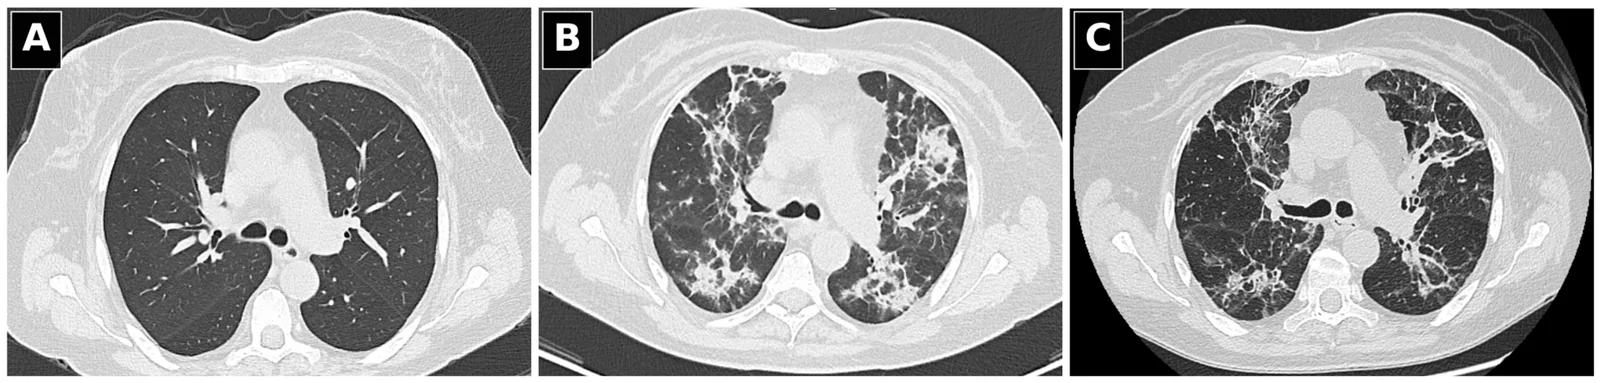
Serial axial chest computed tomography at the level of the tracheal bifurcation, lung window. (A) On 28 July 2025, before starting imatinib: normal lung parenchyma, with no interstitial or airspace abnormality. (B) On 30 December 2025, approximately five months after starting imatinib and one month after symptom onset: extensive bilateral peribronchovascular consolidative opacities with irregular margins, predominantly in the upper lobes, without pleural effusion, in an organizing-pneumonia-like distribution. (C) On 11 February 2026, at the time of acute respiratory failure: coarse reticulation with interlobular and intralobular septal thickening, traction bronchiectasis and architectural distortion superimposed on ground-glass attenuation, indicating evolution towards a fibrosing pattern.
